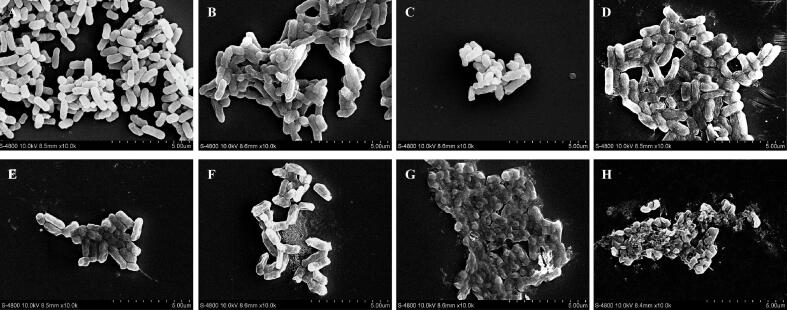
FESEM-based observations (10,000 × magnification) of untreated *Salmonella* (A) and *Salmonella* treated with ultrasound (230 W/cm^2^) for 6 min (B), and *Salmonella* treated with ultrasound (345 W/cm^2^) for 6 min (C), and *Salmonella* treated with ultrasound (345 W/cm^2^) for 9 min (D), and *Salmonella* treated with LEON at 0.08 μL/mL for 6 min (E), and *Salmonella* treated with LEON + US (230 W/cm^2^) for 6 min (F), and *Salmonella* treated with LEON + US (345 W/cm^2^) for 6 min (G), and *Salmonella* treated with LEON + US (345 W/cm^2^) for 9 min (H).
